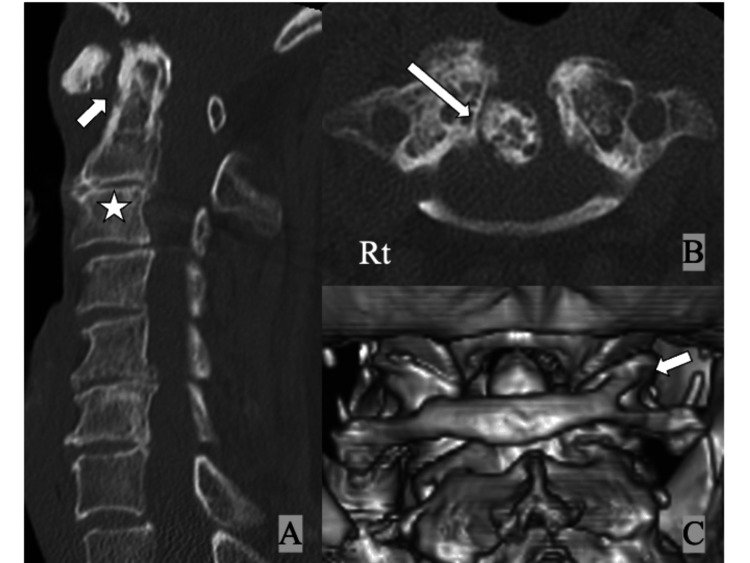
CT of the cervical spine at initial presentation showing (A) increased atlantodental interval (white arrow) and spontaneous fusion of the axis and C3 (white star), (B) osteoarthritic change with bony spur around the dens (white long arrow), and (C) the bony bridge between the posterior arch and the superior facet of the atlas on the right side (complete "ponticulus posticus", white arrow).
